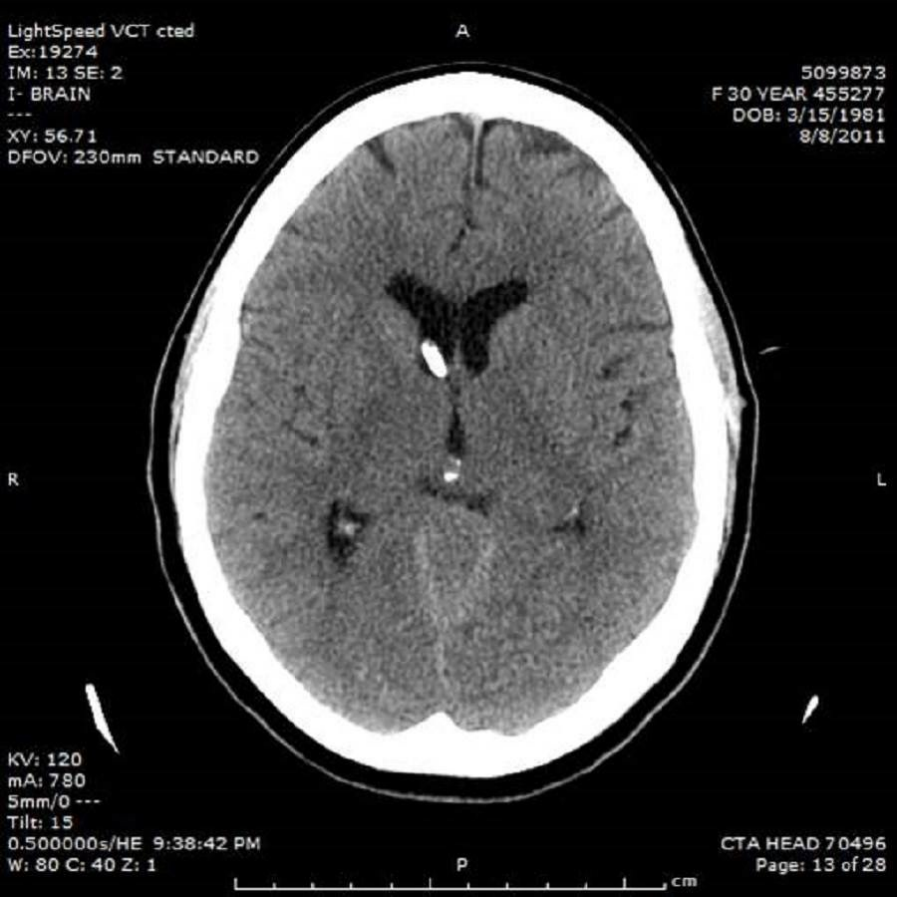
CT of the head revealing placement of a right frontal external ventricular drain for acute obstructive hydrocephalus.
